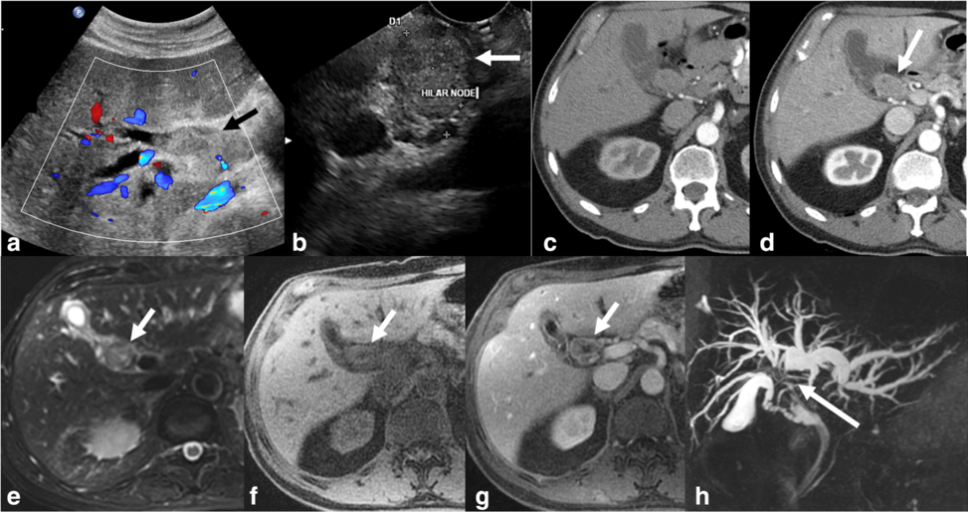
CCA of common hepatic duct with involvement of confluence. Ultrasound with color flow overlay (a) showing an echogenic mass (arrow) filling the common hepatic duct with upstream dilatation of the intrahepatic ducts. EUS image (b) showing a hilar lymph node and was sampled positive for carcinoma. Contrast enhanced CT in arterial phase (c) and portal venous phase (d) showing enhancing mass (arrow) within the duct and no major vascular involvement. The ductal mass is hyperintense on T2-weighted image (e) and hypointense on T1-weighted image (f) and shows concentric post contrast enhancement (g). MRCP (h) shows the confluence invasion and non- visualization of the common hepatic duct.
